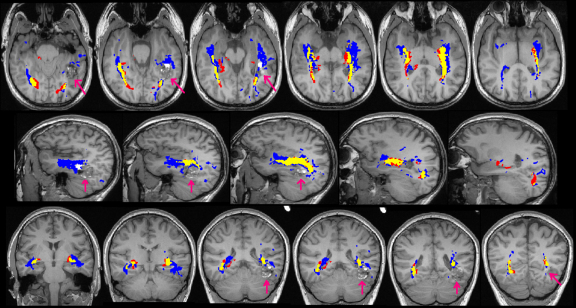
Optic radiation identified in patient 2 by Method 1 only (blue), Method 2 only (red) and both methods (yellow) overlaid on the T1-weighted image. The arrows indicate the left temporal cavernoma. Image conventions as Fig. 3, with the coronal images being from anterior to posterior.
